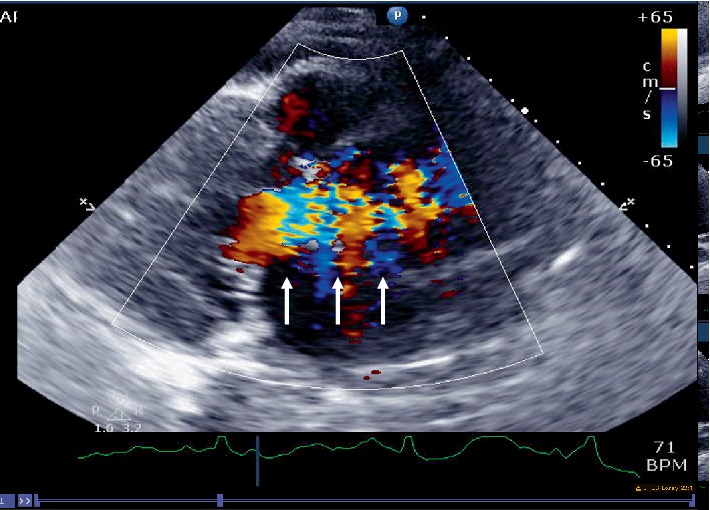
Parasternal long axis view showing aortic insufficiency (arrows).
